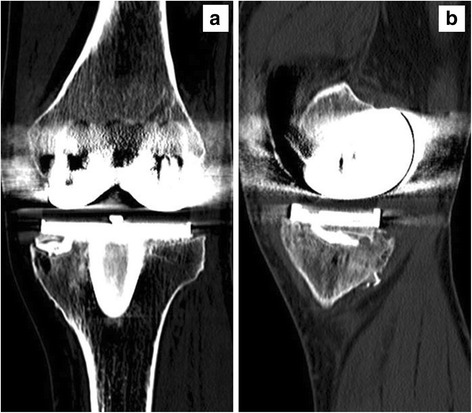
a Postoperative anteroposterior and (b) lateral CT scans at 1 year, demonstrating autograft incorporation with cross trabeculation between the proximal tibial bone and graft
